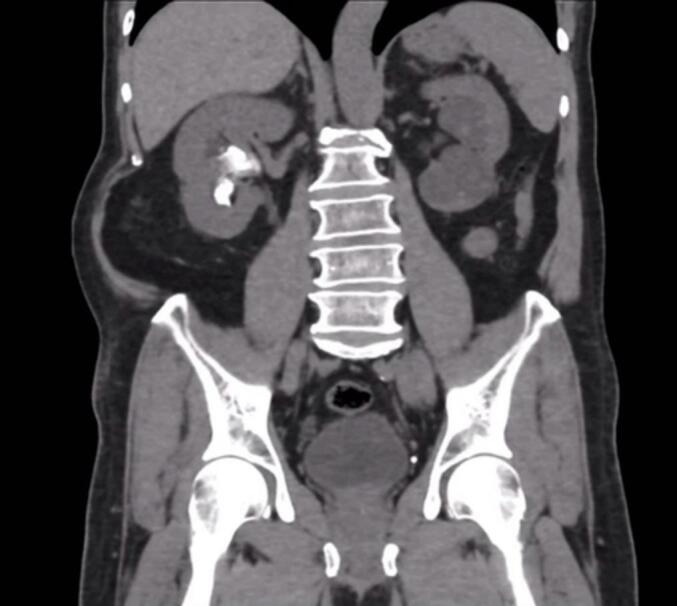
Preoperative CT scan showing a right staghorn stone.
